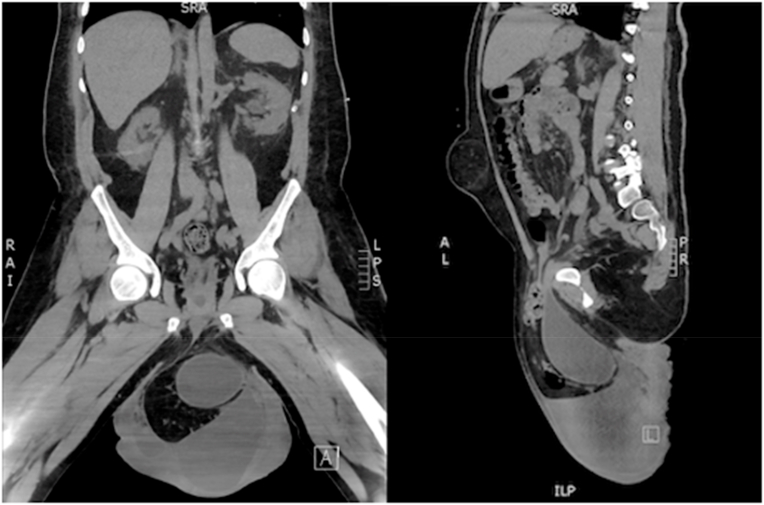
CT-scan before surgery, showing majority of the bladder, a part of the sigmoid colon and some of the omentum situated in the left inguinal hernia. Besides the above, the patient had two big hydroceles, and bilaterally there were hydronephrosis and hydroureter, which were immediately relieved with two nephrostomy catheters. The scan also shows an umbilical hernia which did not represent any threat to the patient and was left untreated.
